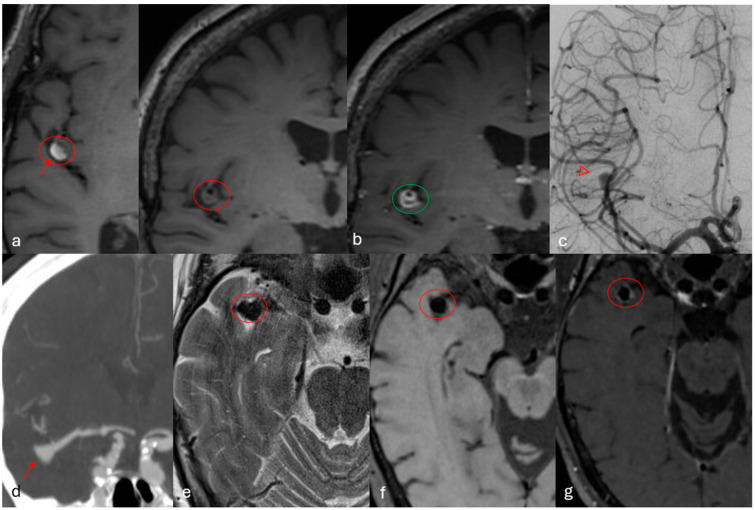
Two cases of patients without large vessel occlusion. (a) In the upper row T1 axial and coronal native images show a partially thrombosed aneurysm at the distal M2 segment of the right MCA (red circle). The thrombosed portion with T1 hyperintensity is depicted by the red arrow. (b) T1 BB with contrast shows vivid enhancement of the aneurysm wall and clot (green circle). The enhancement is evaluated as vivid because it is intense, well demarcated and close to that of normally enhancing intracranial structures (c) Angiographic anteroposterior images show partial contrast filling of the patent part of the sac (open red arrowheads). This case was considered to have a high probability of the aneurysm being responsible for ischemia. The aneurysm was treated surgically with clipping. The second case is depicted in the lower row. (d) A CTA shows an inferiorly oriented right MCA bifurcation aneurysm (red arrow). MR imaging with axial (e) T2, (f) T1 BB and (g) T1BB with contrast show a non-thrombosed aneurysm with light contrast enhancement (red circle). The enhancement is evaluated as light because it is subtle, slightly above background parenchyma. The patient was diagnosed with atrial fibrillation, had additional multiple older infarcts in different vascular territories and the cause of the newer ischemic lesions were considered to be unrelated to the aneurysm.
